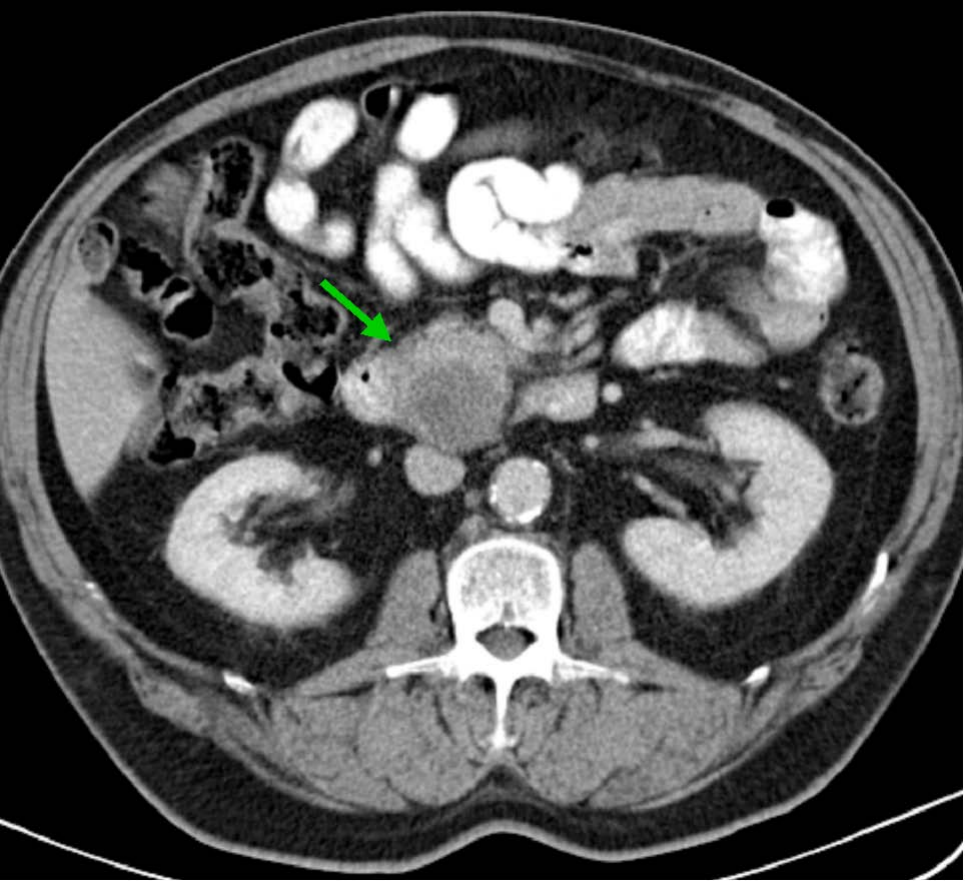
Undifferentiated carcinoma, sarcomatoid variant of the head of the pancreas: computed tomography scan of the abdomen shows a lesion of the head of the pancreas head with cystic and solid parts.
